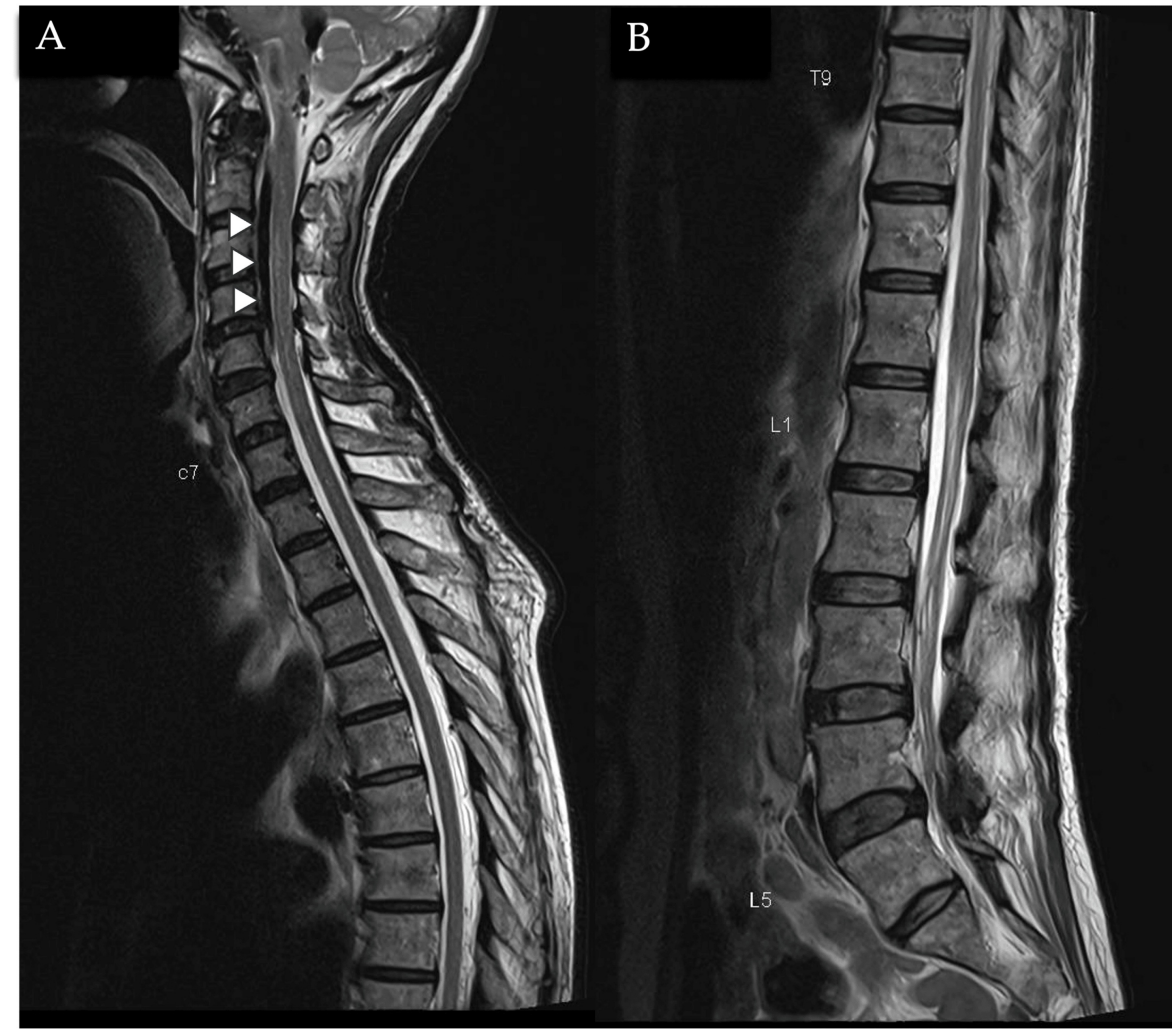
MRI whole spine T2 sequences in sagittal cuts of the cervicothoracic spine (4A) and thoracolumbar spine (4B), showing the presence of air within the epidural space of the cervical canal (white arrowheads), which appears as linear low T2 signal within the epidural space. There is no significant abnormality at the site of the lumbar puncture.
